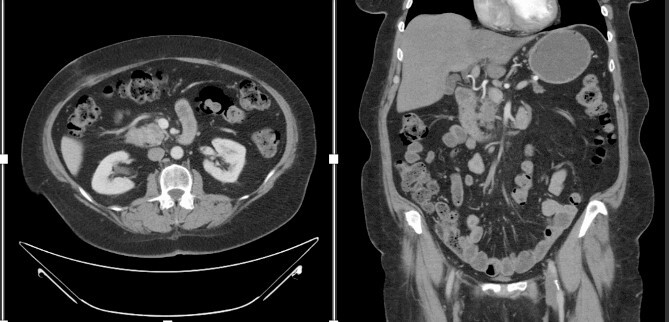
CT abdomen and pelvis with IV contrast with axial and coronal views demonstrating the Pancreatic mass.
